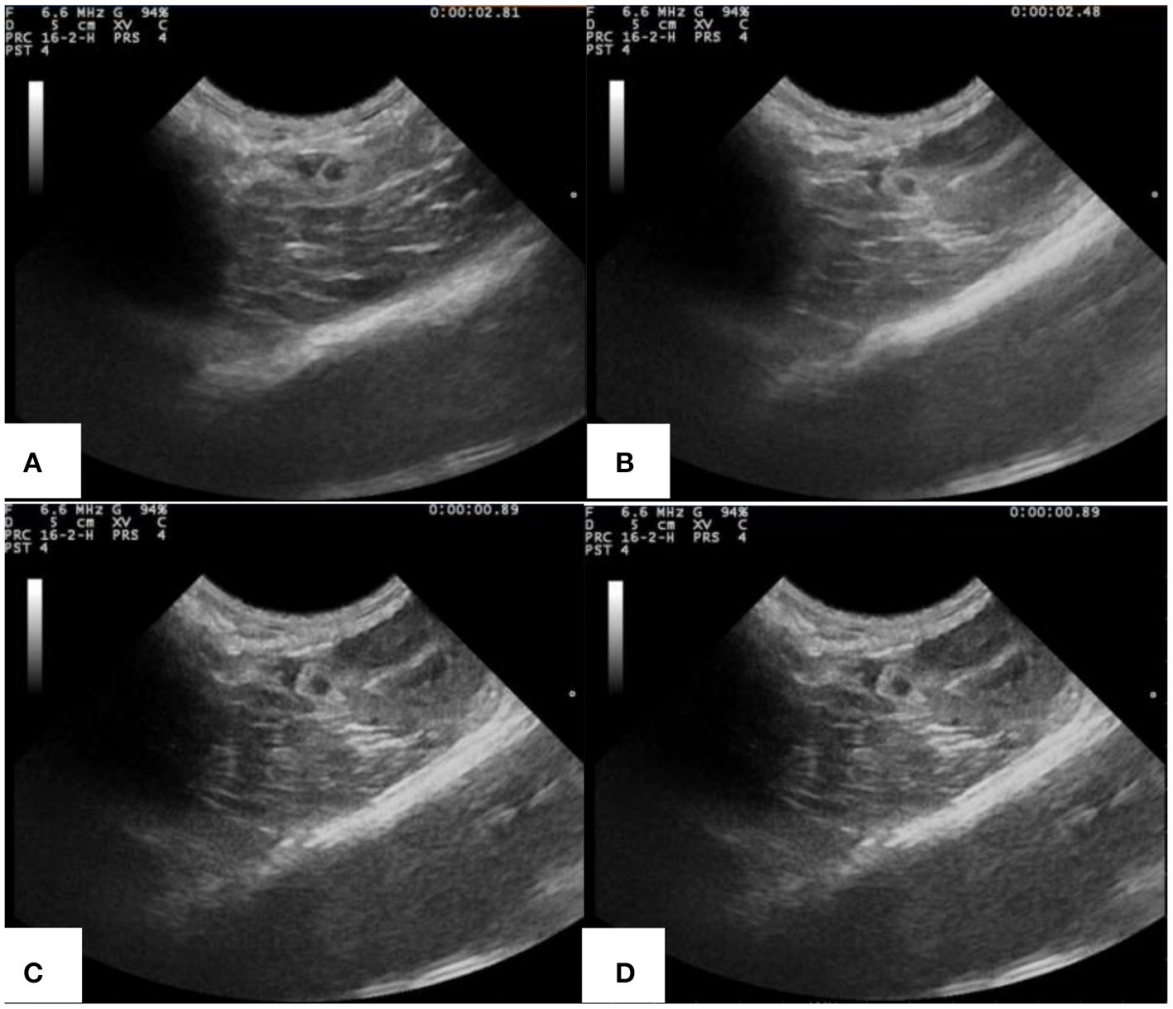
Ultrasonic imaging of rabbit abdominal aorta of rabbits injected with PLA combined Fe_3_O_4_-GO-ASA nanobubbles of the different concentrations (A) 0 mg·mL^−1^
